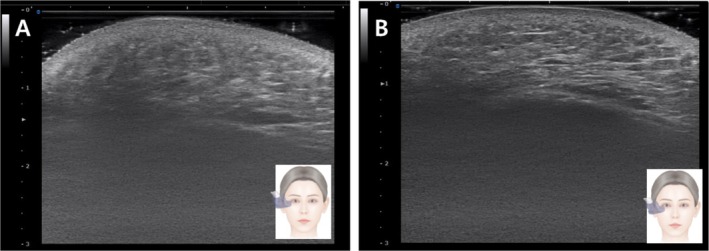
Horizontal ultrasonographic image of the zygomaticus ligament proper before (A) and after (B) treatment.
